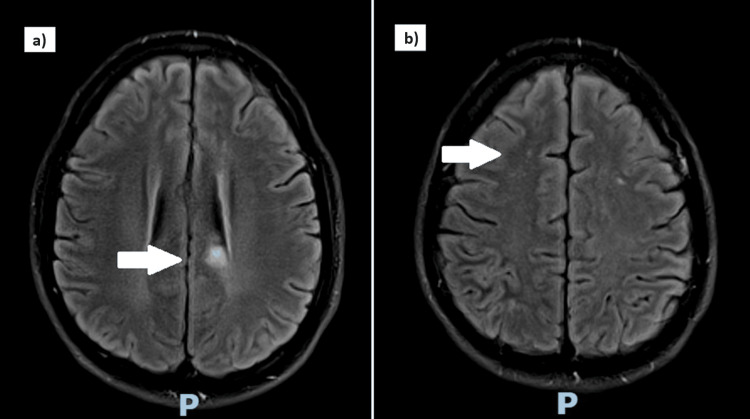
Diffusion-weighted images of the brain MRI show acute multifocal infarcts in a) the left aspect of the splenium of the corpus callosum (arrow) and b) the right parietal subcortical white matter with no intra- or extra-cranial stenosis (arrow).
